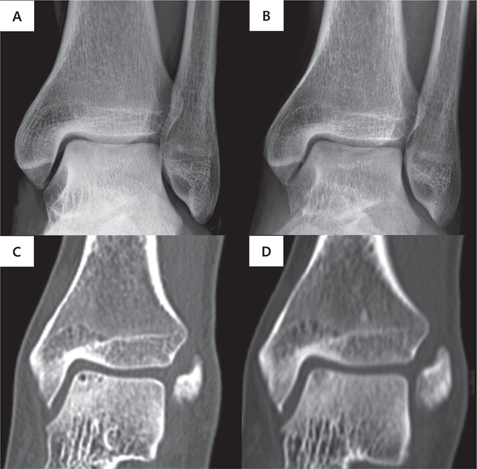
A 53-year-old man with OCL. (A) Initial standing radiograph and (C) CT. (B) Standing radiograph and (D) CT at 7-year follow-up. The lesion size decreased, and the AOFAS ankle–hindfoot score improved from 73 to 100.
